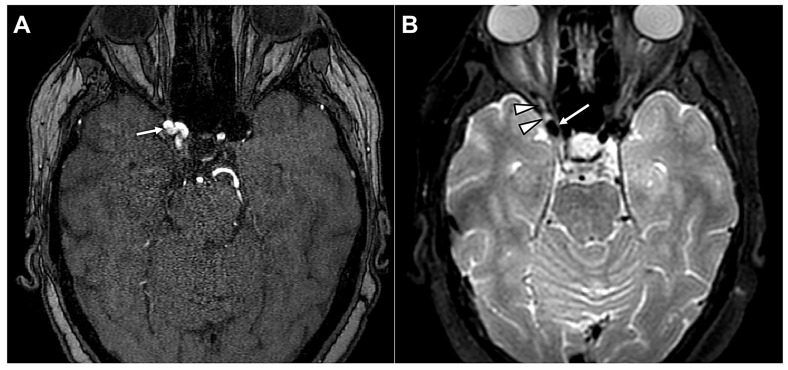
MRI findings of a supraclinoid internal carotid artery (ICA) aneurysm with secondary oculomotor nerve (OMN) compression. (A) Axial Time-of-Flight (TOF) MR angiography demonstrates a saccular aneurysm arising from the supraclinoid segment of the ICA, projecting laterally (arrow). (B) Corresponding axial T2-weighted imaging reveals thickening and hyperintensity of the adjacent OMN (arrowheads). The findings were compatible with edema secondary to mass effect from compressive neuropathy due to the aneurysm (arrow). The patient’s symptoms included third nerve palsy and pupillary dilation. Image Credit: Dr. Nikolaos-Achilleas Arkoudis and Prof. Georgios Velonakis.
